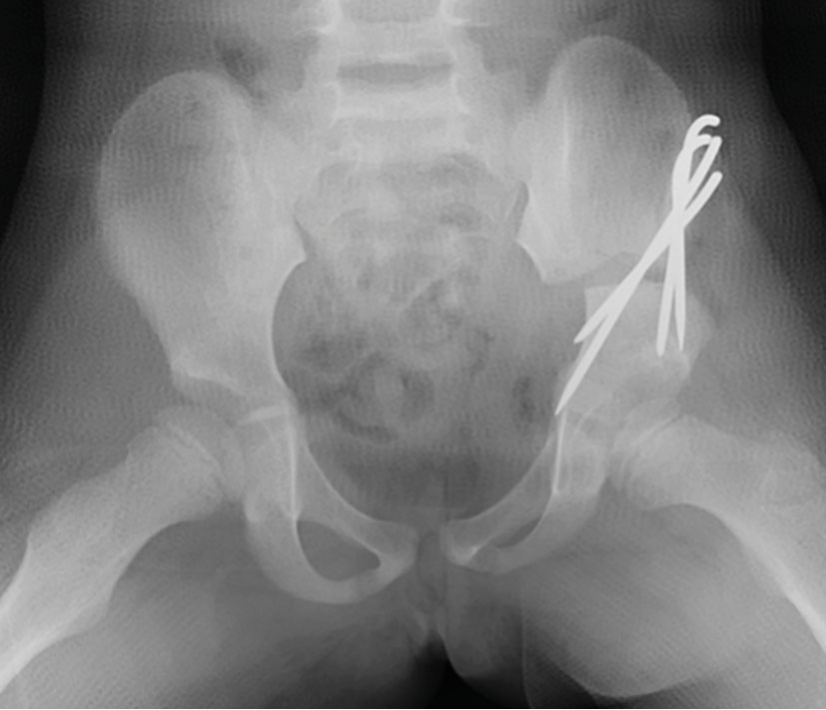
Radiograph of the hips just after Salter innominate osteotomy.
